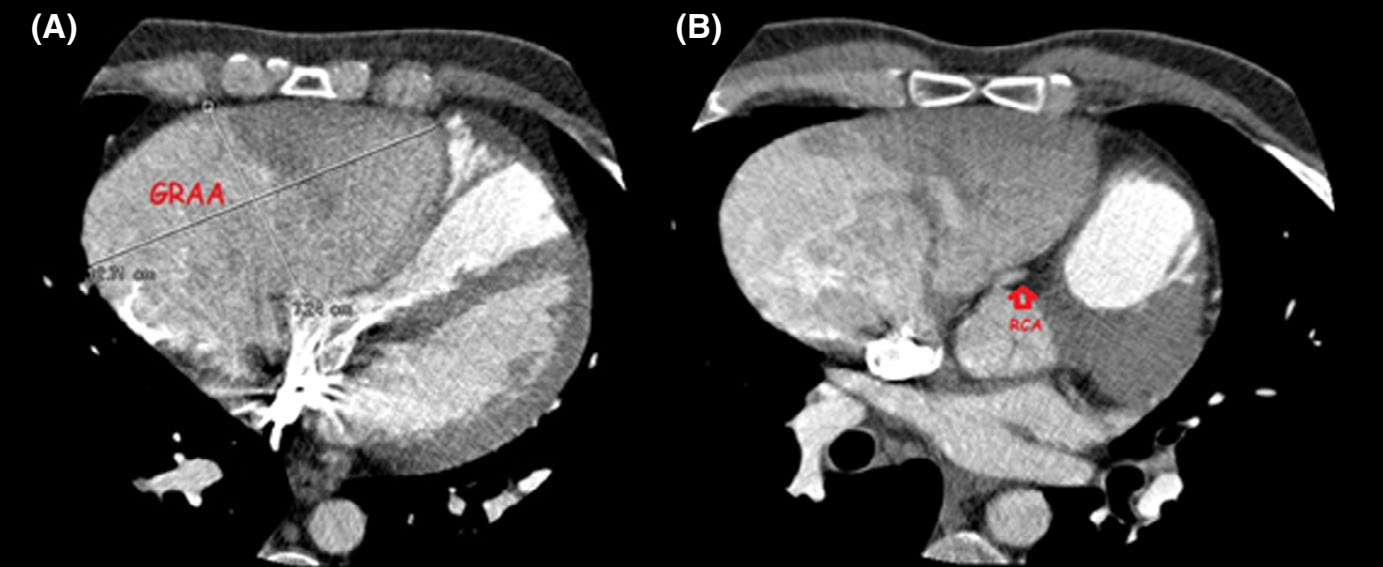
(A) (Left) Spiral CT‐ angiography was performed. A GRAA measuring approximately 127*122*72 mm is depicted in the anterior and right aspects of the cardiac chambers, just in front of the right atrium and right ventricle.^2^ (Right): Due to the compressive effect of the GRAA, the RCA and its branches and in particular, the SA‐ nodal branch, are displaced leftward.GRAA: Giant right atrial aneurysm, RCA: right coronary artery, SA node:Sinoatrial node
